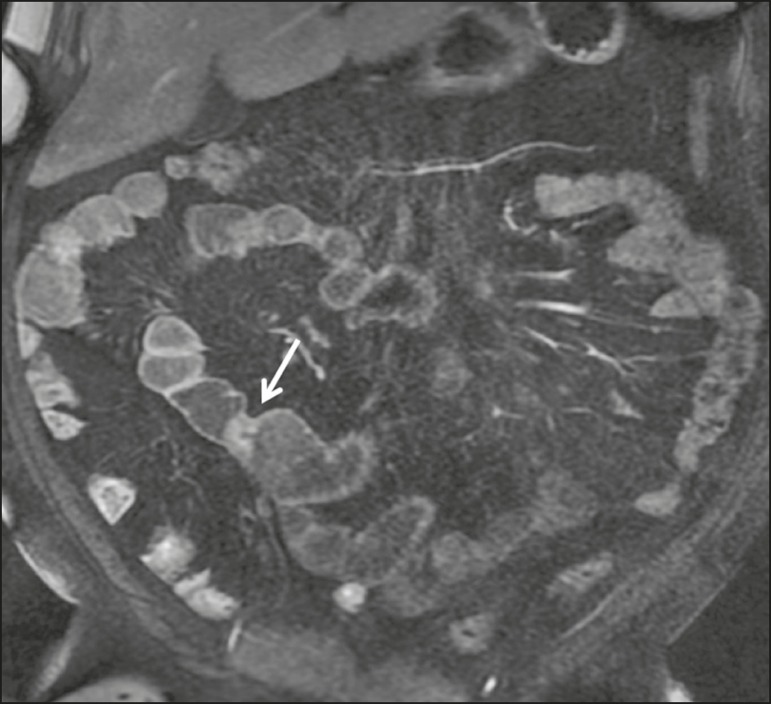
Coronal, paramagnetic contrast-enhanced (gadolinium), fat saturated T1-weighted MRE sequence showing wall thickening with focal stenosis (arrow) and dilatation of the prestenotic segment.
